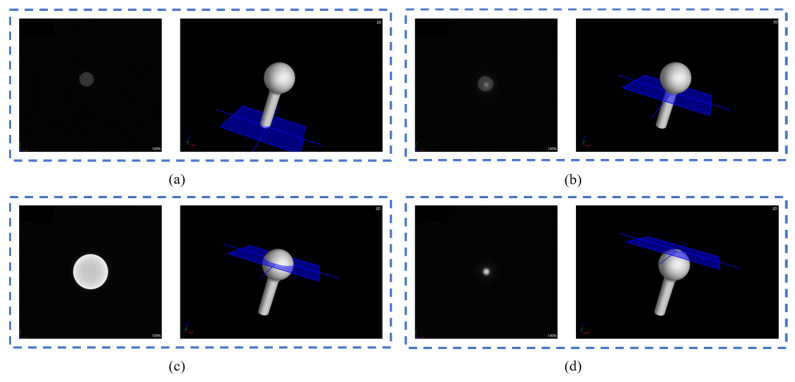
Slices of cylinder-ball reference object CT reconstructed volume data (slice order: a–d).
